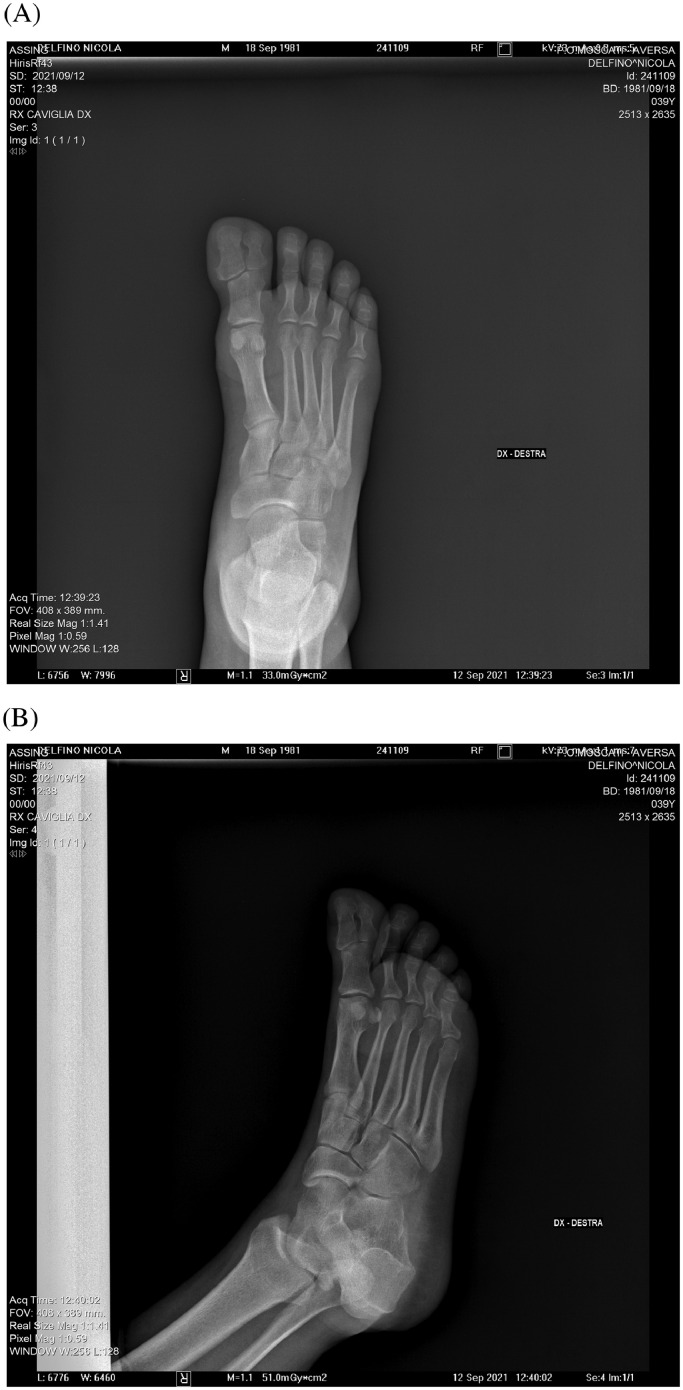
Anterior posterior (A) and laterolateral (B) radiograph of the right foot showing the distal phalangeal type of preaxial polydactyly.
